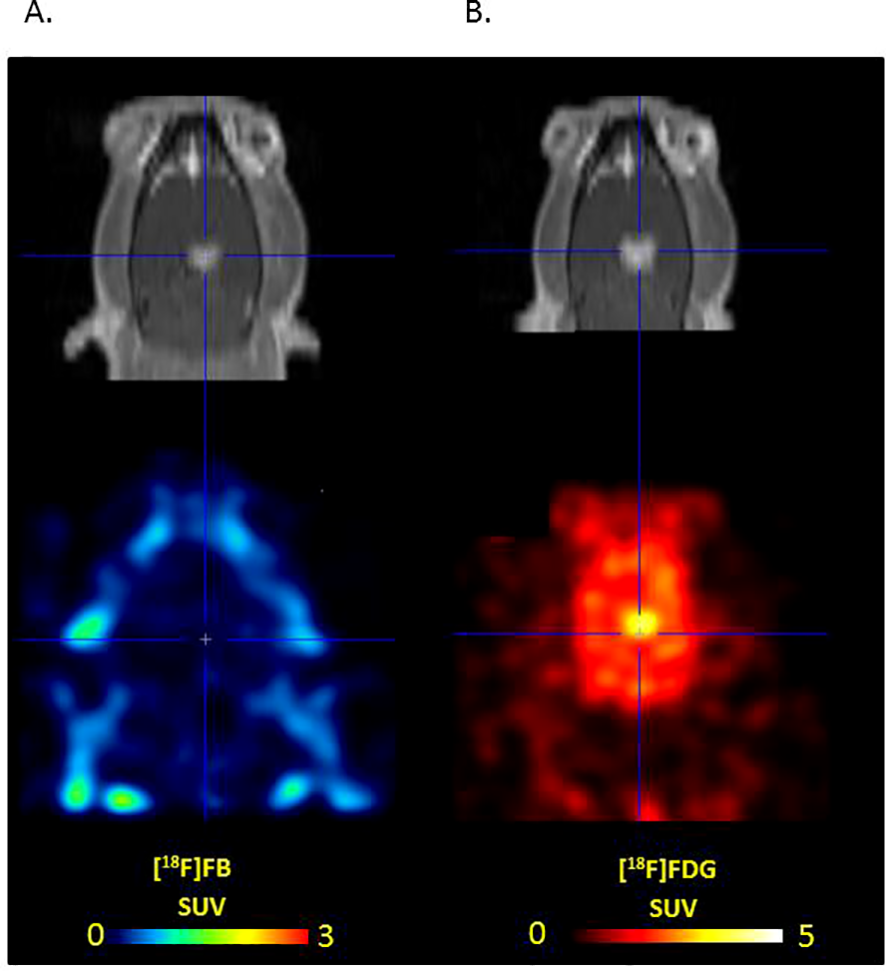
Unconjugated label uptake. T1 –MRI image with contrast enhancement and (a) [^18^F]-fluorobenzylazide and (b) [^18^F]FDG. While [^18^F]FB demonstrates no uptake, [^18^F]FDG accumulates in the lesion.
